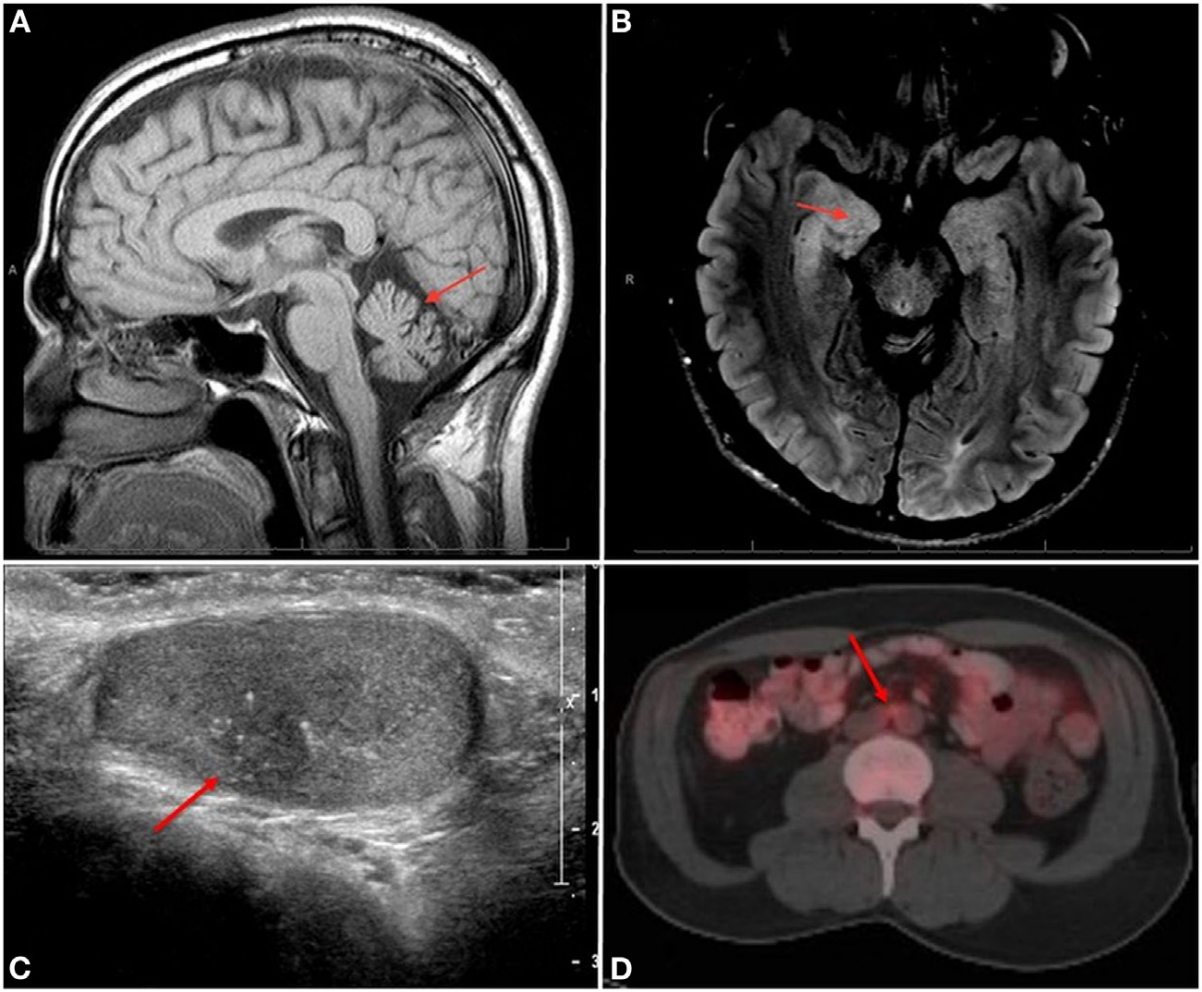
(A) Sagittal T1 image shows vermian atrophy (arrow) and (B) axial FLAIR image shows right hippocampal hyperintensity (arrow). (C) Right testicular US showing a 9 mm × 7 mm hypoechoic lesion (arrow) with microcalcifications. (D) F18-FDG positron emission tomography/computerized tomography showing an FDG-avid 1.4-cm × 0.9-cm lymph node in the periaortic chain (arrow).
